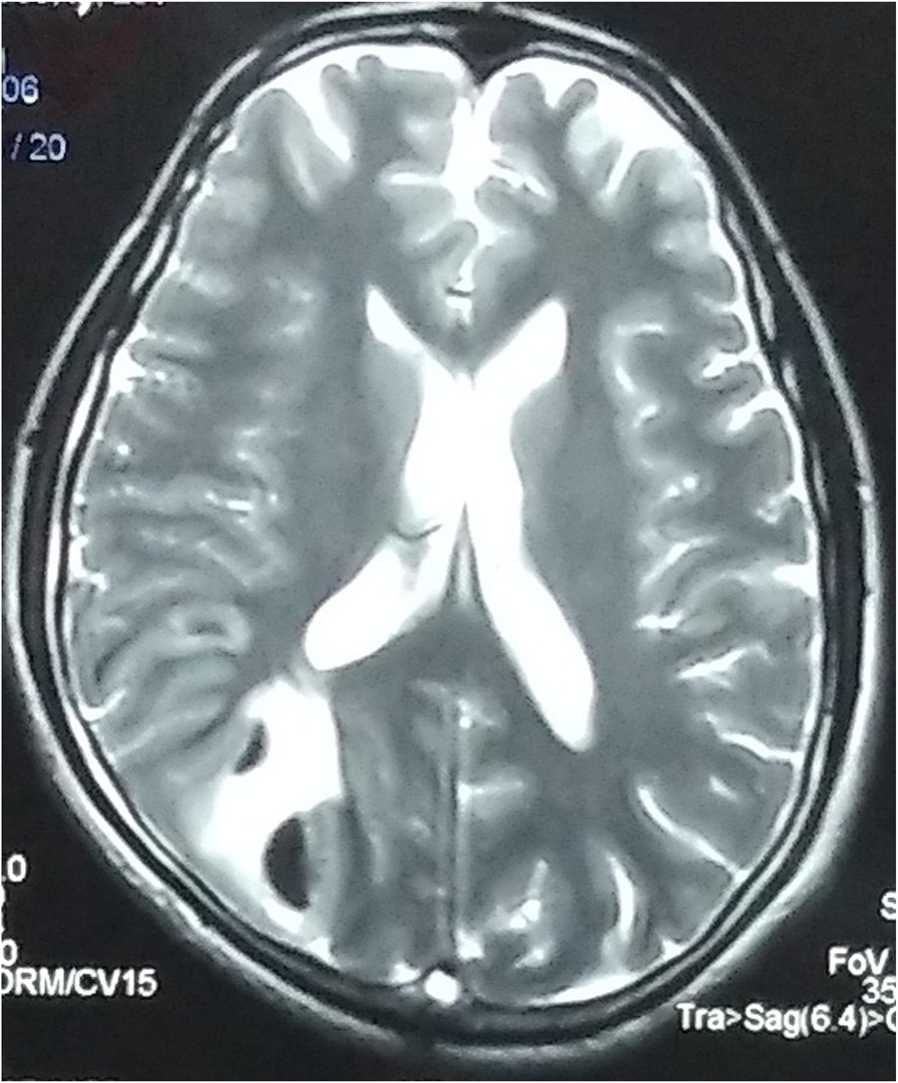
T2-weighted axial images of the brain show mixed signal intensity lesion in right posterior parieto-occipital lobe with areas of hypointensity
